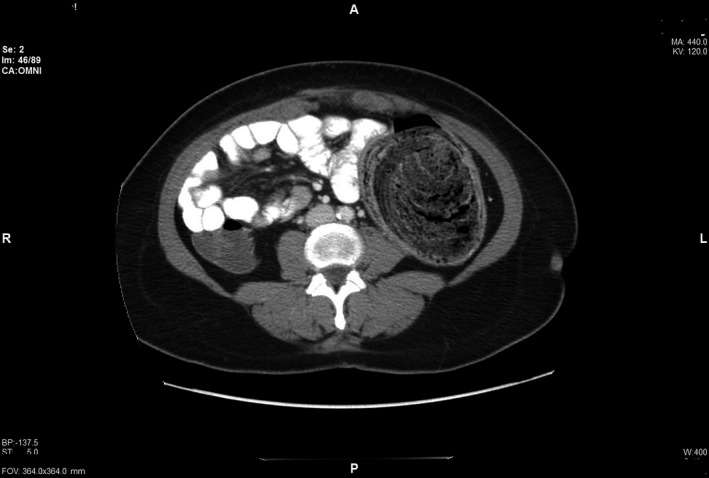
demonstrates cross section of the CT scan of abdomen with the large fecaloma
